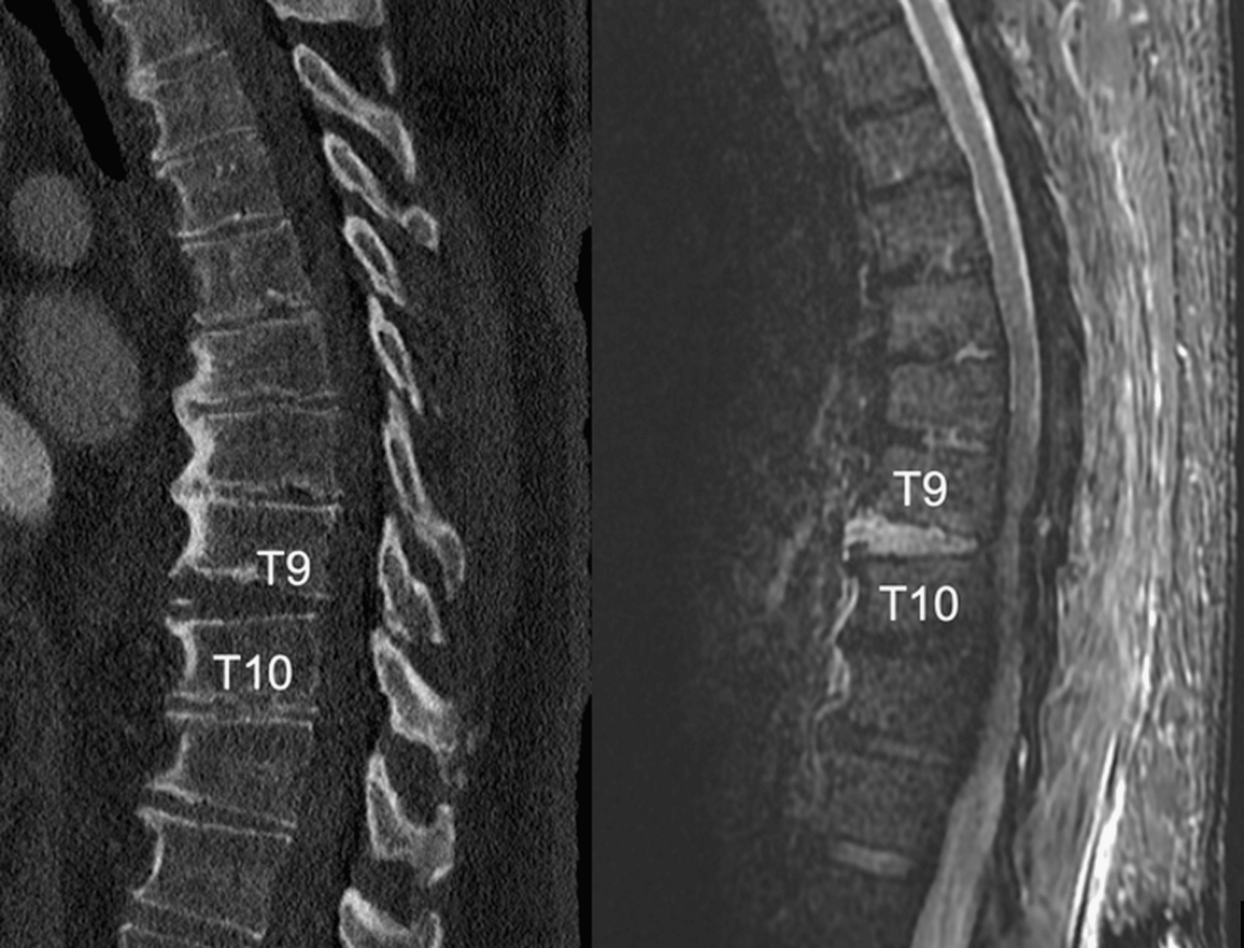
A 72-year-old male with DISH and a T9-T10 hyperextension fracture seen on CT and MRI. DISH = diffuse idiopathic skeletal hyperostosis
